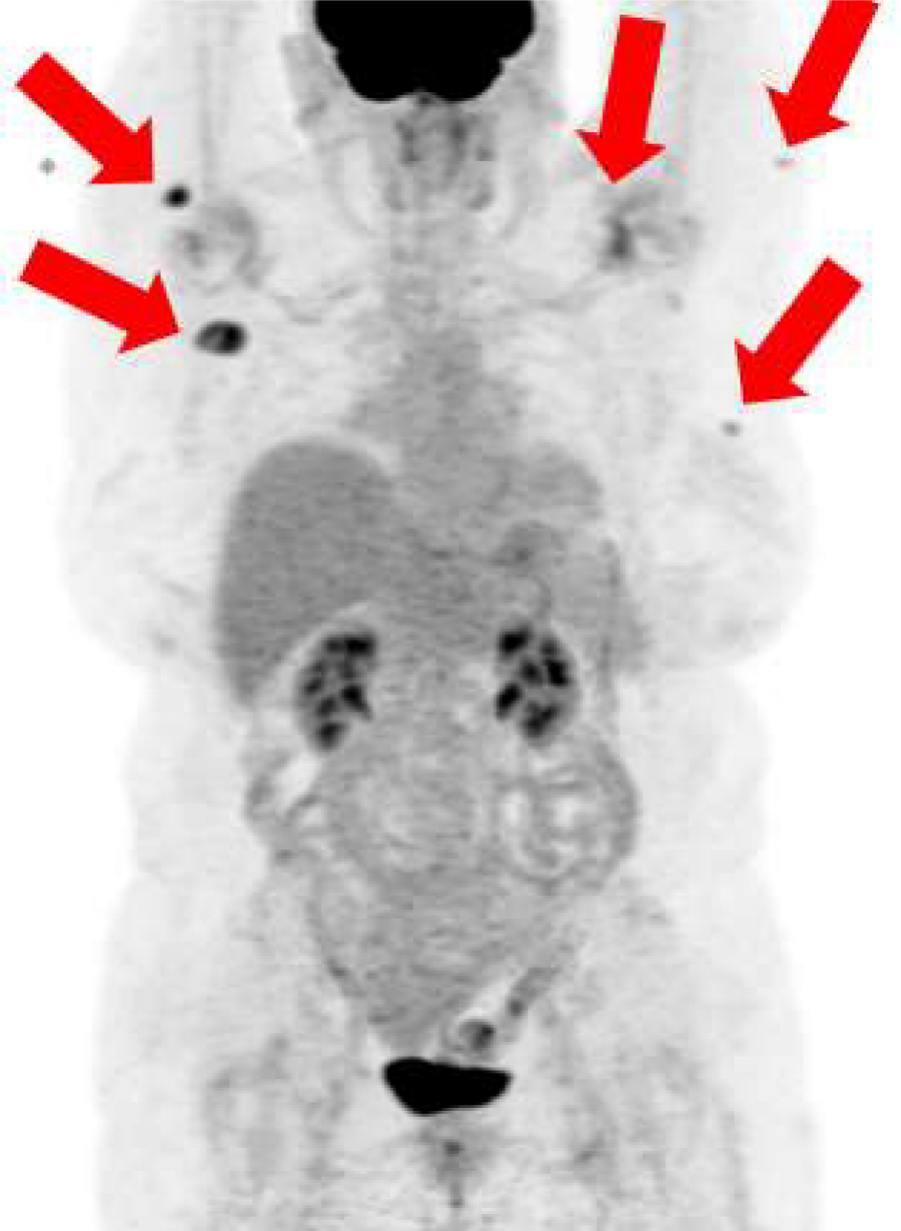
PET/CT demonstrated numerous suspicious lesions, including the left breast (SUV 1.9), left proximal arm laterally (SUV 2.1), left back laterally (SUV 2.8), proximal right arm/right shoulder posteriorly (SUV 7.2/1.1 cm), right back posteriorly– not imaged (SUV 1.8), and right back laterally posterior to the right scapula with clustered lesions (SUV 7.6).
